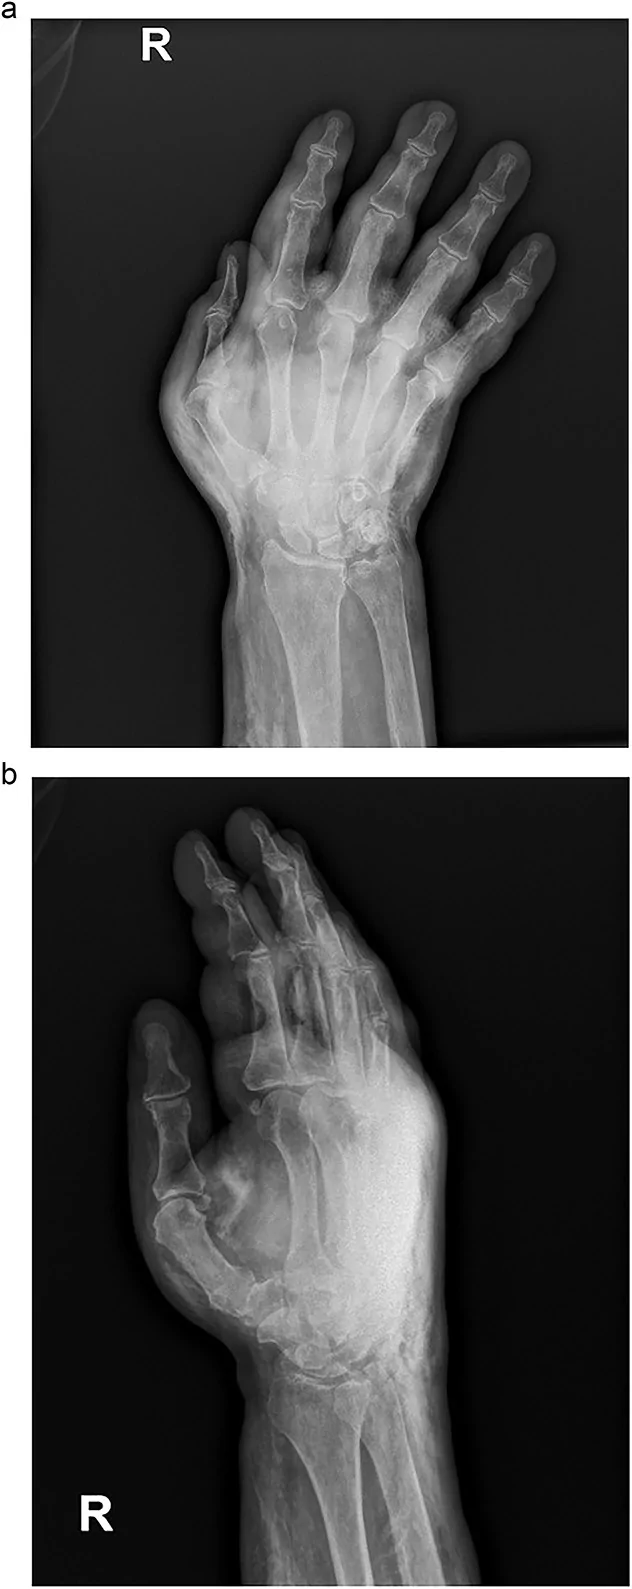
(a, b) X-ray of the right hand: Extravasation of contrast media into the soft tissues.
